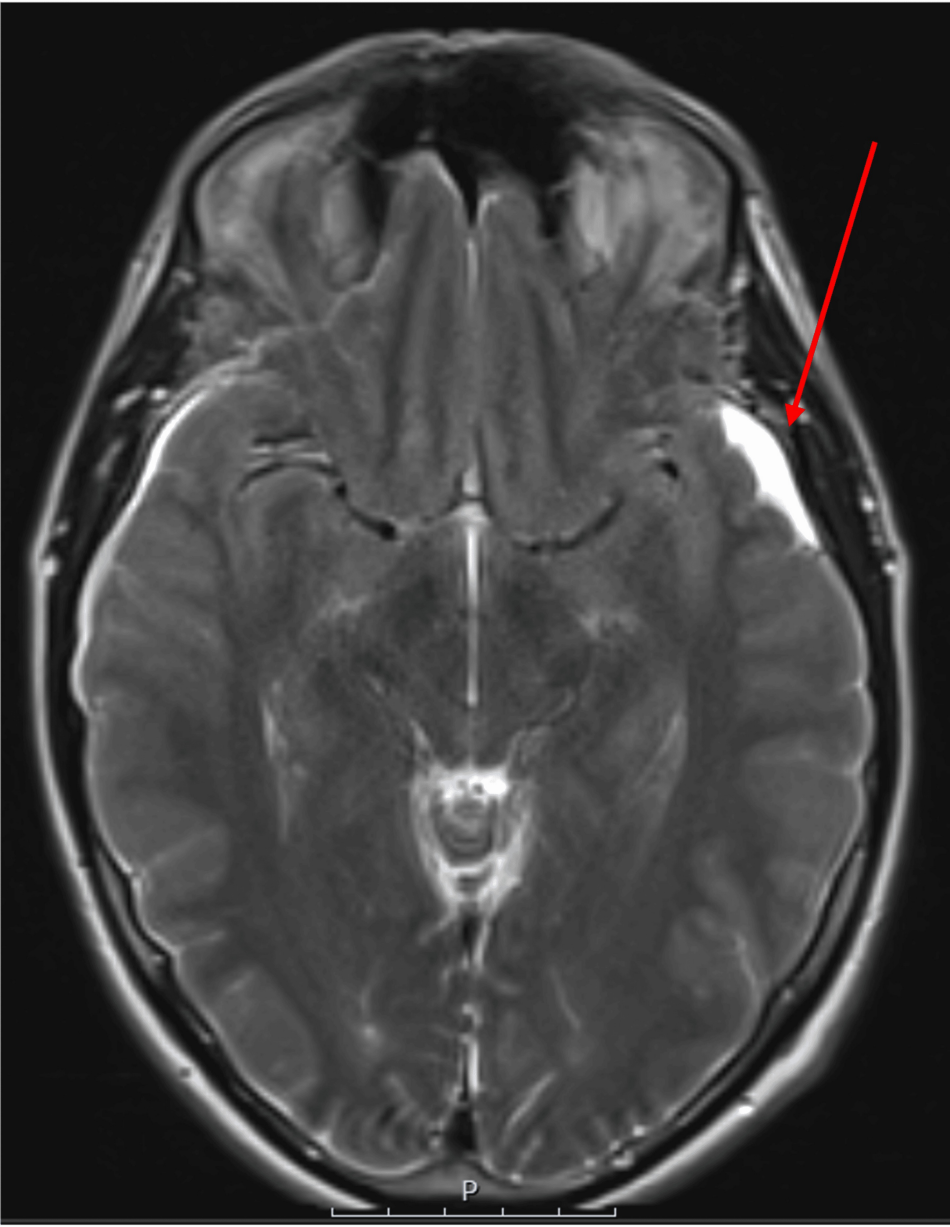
MRI of the head showing bilateral shallow subdural hematomas
